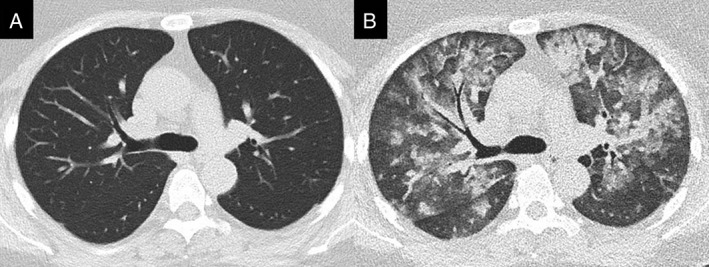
The chest computed tomography (CT) findings: (A) low‐dose computed tomography of health check‐up showed normal attenuation of bilateral lung parenchyma; (B) repeated CT scan 4 h later revealed multiple ground glass attenuation and airspace consolidation in bilateral lungs.
